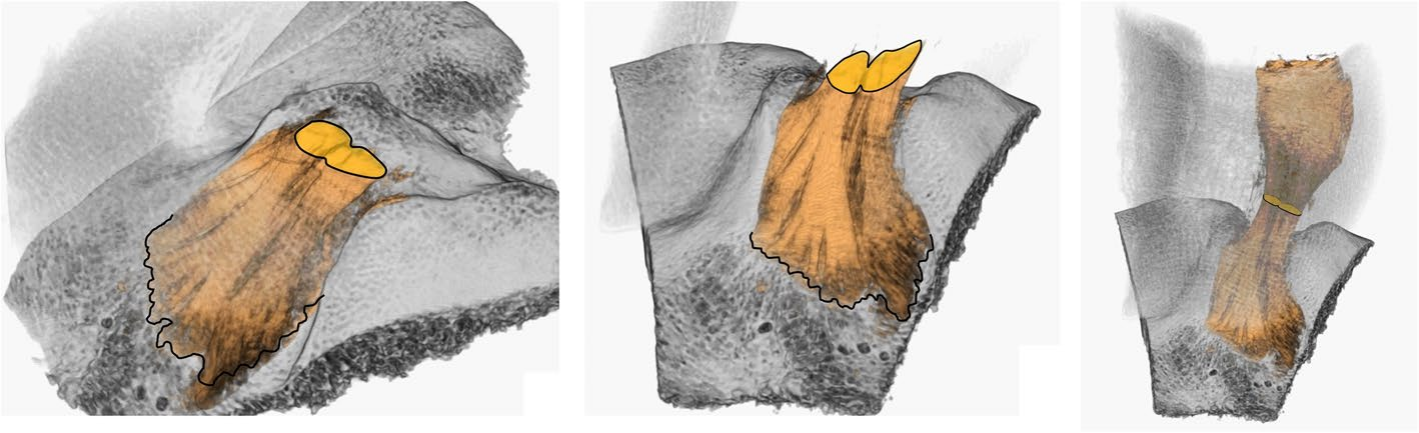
3D-CT of the tibial insertion of a human ACL
